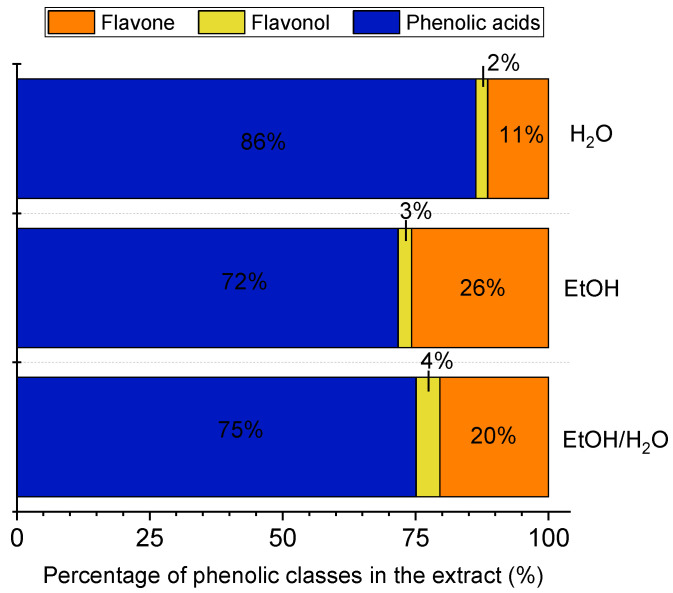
Percentage of the phenolic classes in the extracts of *Eryngium foetidum* leaves as obtained by ultrasound-assisted extraction using water (H_2_O), ethanol (EtOH), or a mixture of ethanol/water (1:1, *v*/*v*) (EtOH/H_2_O) as green solvents.
